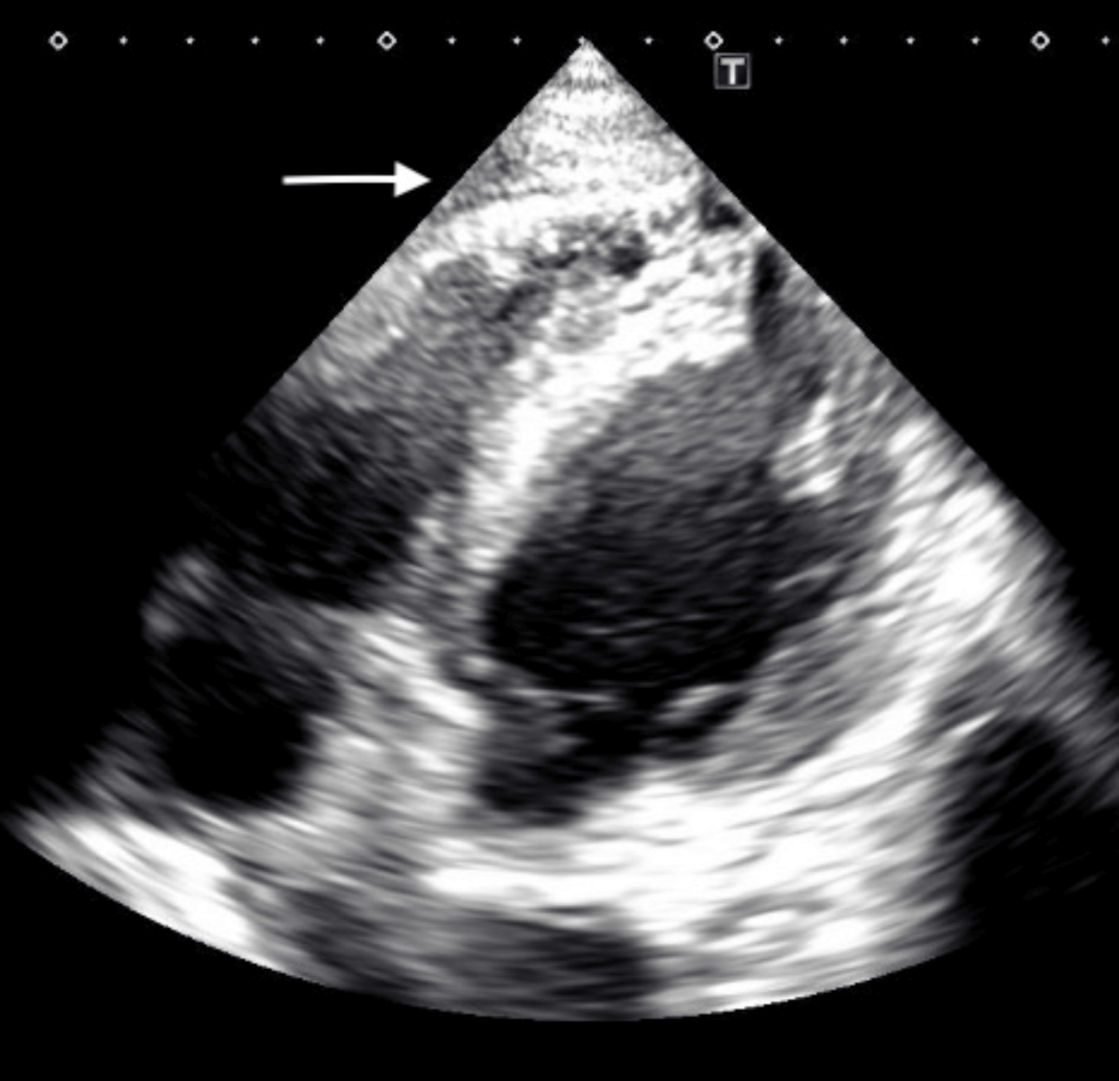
Transthoracic echocardiography after pericardiocentesis revealing a small amount of pericardial fluid (arrow).
